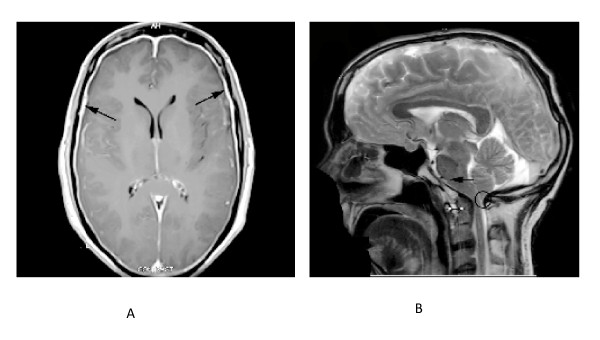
MRI Brain. a. Axial T1 weighted post contrast image; significant meningeal enhancement (black arrows) suggestive of pachymeningitis is shown. b. Sagittal T2 weighted image; showing characteristics of Spontaneous Intracranial Hypotension: flattening of pons (white arrow) and inferior orientation of cerebellar tonsils (encircled). Atlanto-axial subluxation (white double arrow), is also demonstrated.
